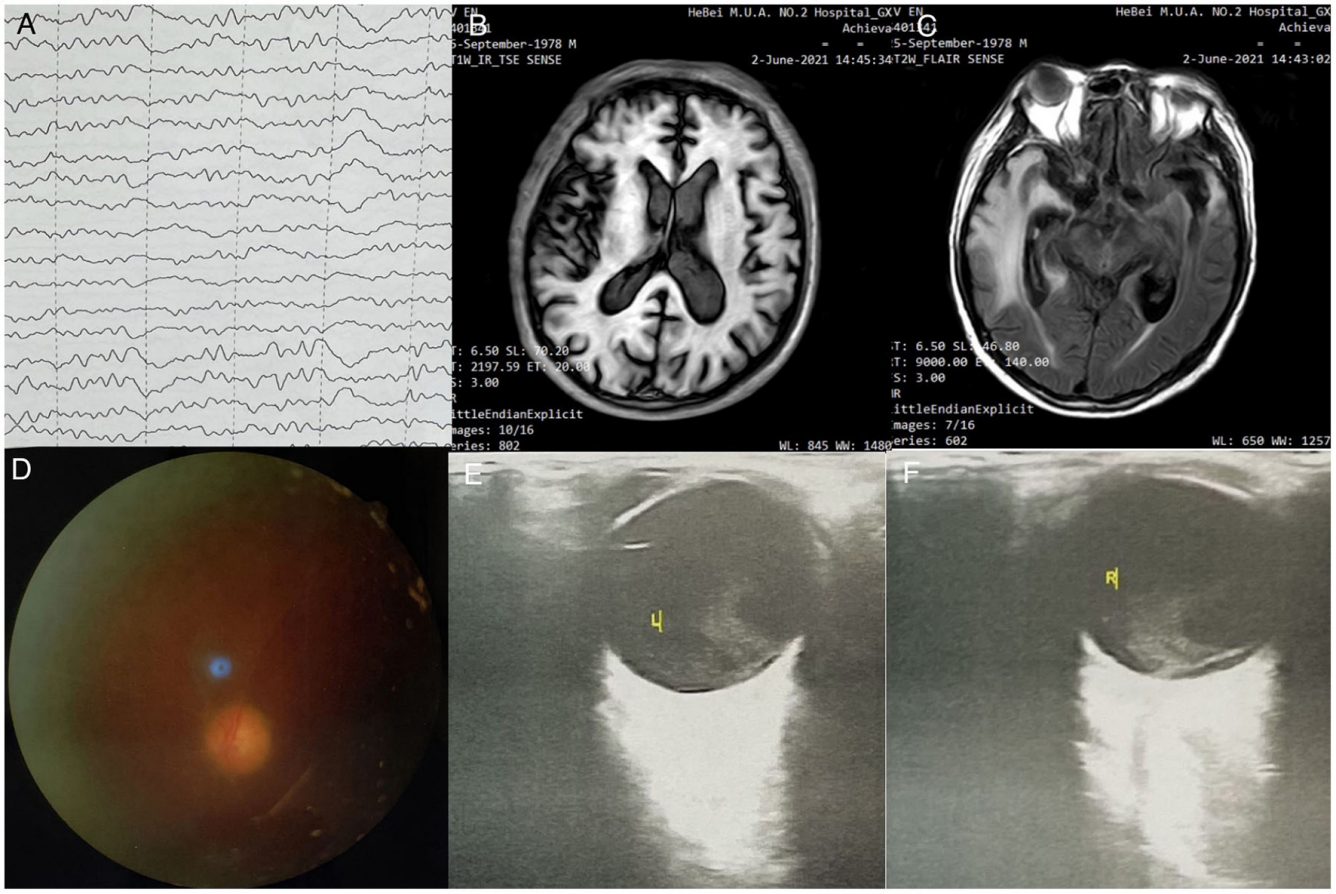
(A) EEG examination: moderate to severe abnormal EEG, background diffuse 0, 6 slow wave bursts, 7-8 Hz slow rhythm bursts in the occipital lobe; (B) Head MRI - T1IR-TSE: normal head size, bilateral frontal lobe, temporal lobe, insula, bilateral hippocampus, bilateral thalamus, bilateral cingulate gyrus, flaky T1 low signal; (C) Head MRI - T2 FLAIR sequence: head size is normal; temporal lobe, insula, and bilateral hippocampus present high signal in T2 FLAIR, high signal in FLAIR; local sulci are clearer than before; (D) Fundus examination-left eye; (E) Ophthalmic ultrasound-L: severe vitreous opacity, mild thickening of the posterior wall of the eyes; (F) Ophthalmic ultrasound-L: severe vitreous opacity, mild thickening of the posterior wall of the eyes, thickening of the inner rectus muscle of the right eye.
